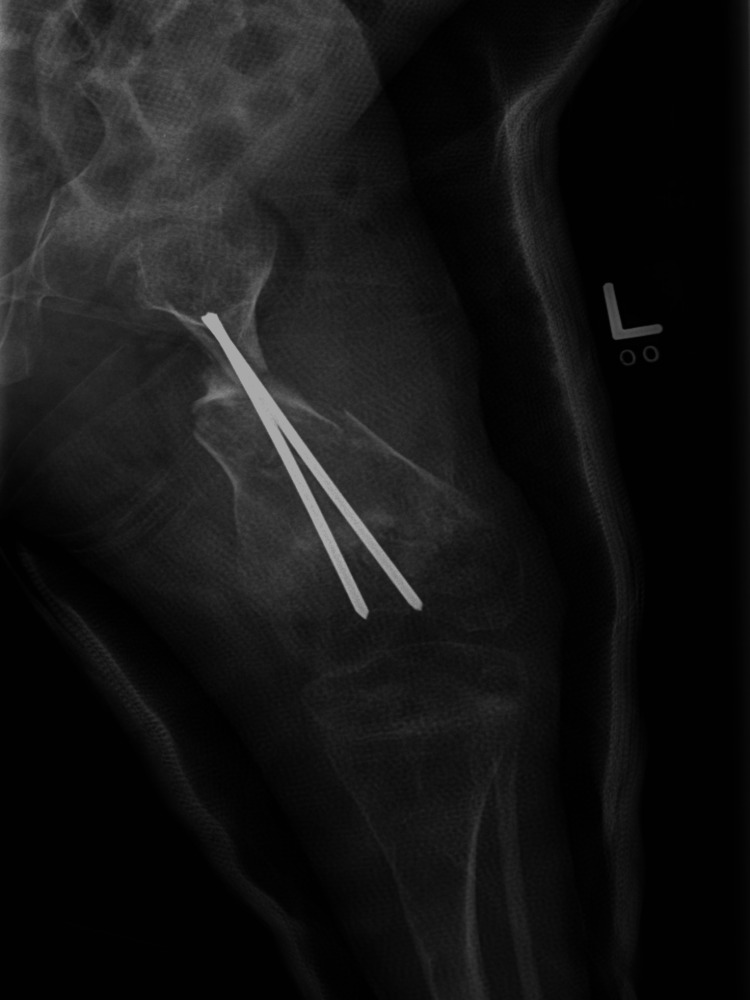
Immediate postoperative X-ray showed left femur osteotomy with rush nail fixation
